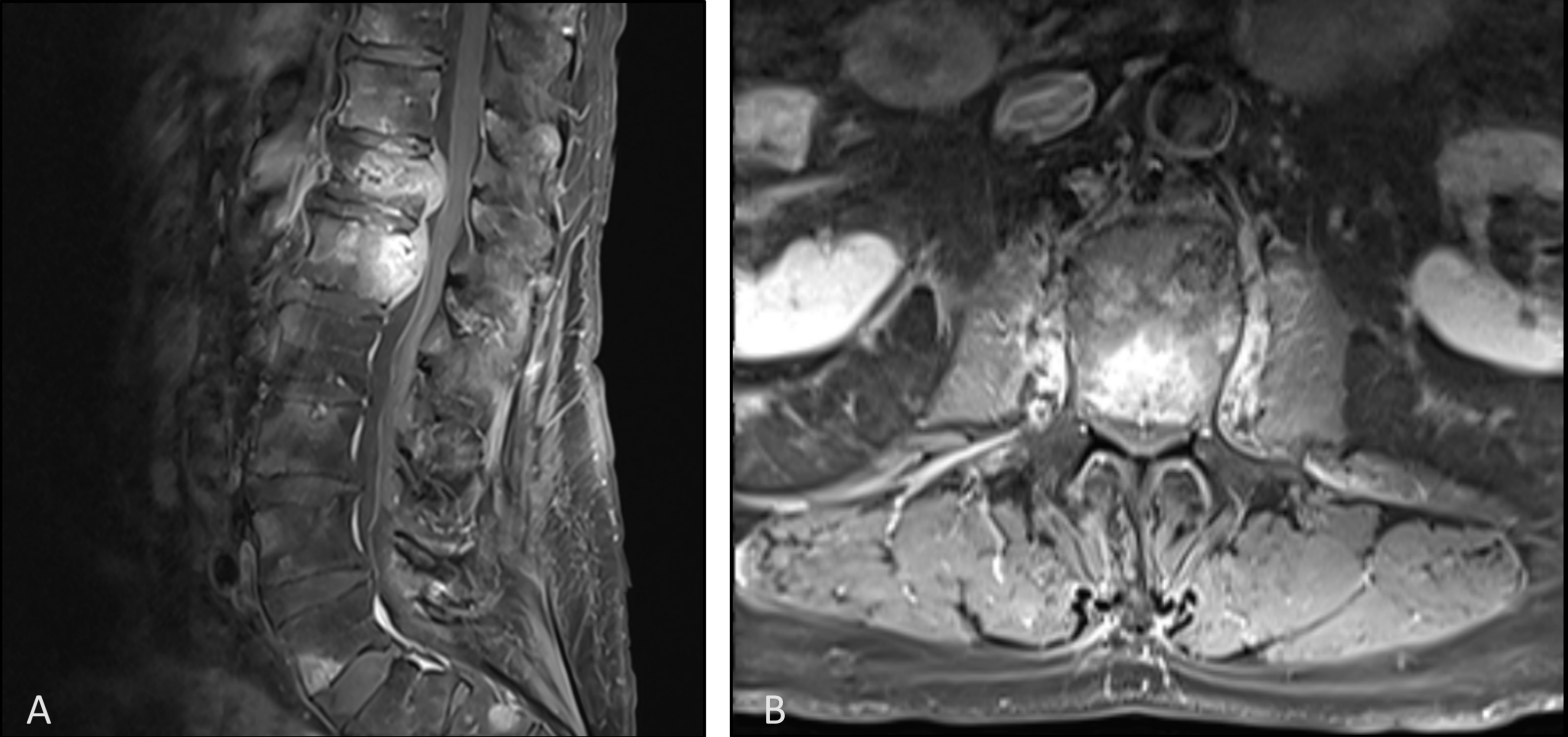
Pre-treatment contrast enhanced T1-weighted magnetic resonance imaging of the sagittal spine (A) and axial spine at L1 (B) showing vertebral metastases and epidural involvement
